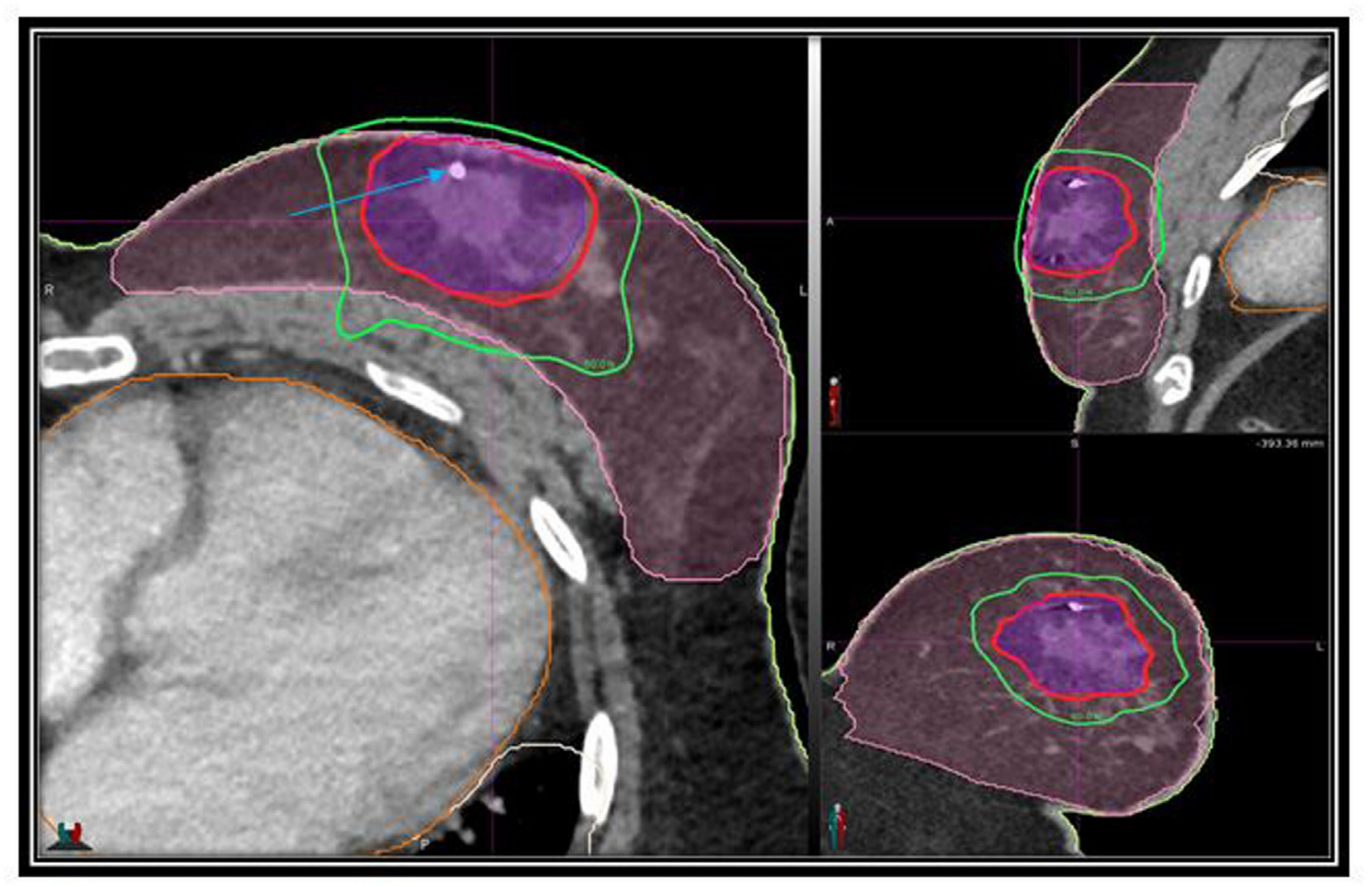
Axial, sagittal, and coronal views of the treatment planning CT scan demonstrating the PTV (purple) and normal breast (pink), isodose lines shown as follows: 100% of the prescription dose, red line; 50% of the prescription dose, green line; arrow points to gold fiducial marker.
